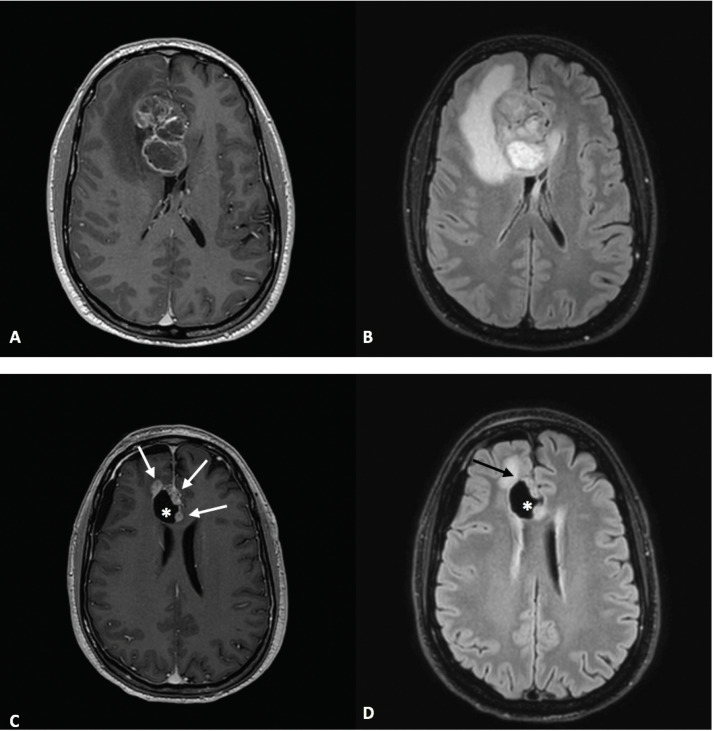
MRI at time of diagnosis and progression. Diagnosis: Axial T1 contrast-enhanced image (A) shows a right parasagittal frontal tumor involving the genu of the corpus callosum. There is predominantly peripheral mural enhancement with central areas of necrotic appearance. It is surrounded by a diffuse hyperintense area on the FLAIR sequence (B), mostly due to edema. Progression: Five months after diagnosis, several enhancing nodules (white arrows in (C)) appear along the anterior and medial margins of the post-surgical cavity (*), suggestive of tumor recurrence. There is scarce edema in the anterior frontal subcortical white matter on the FLAIR image (black arrow in (D)). * refers to post-surgical cavity.
